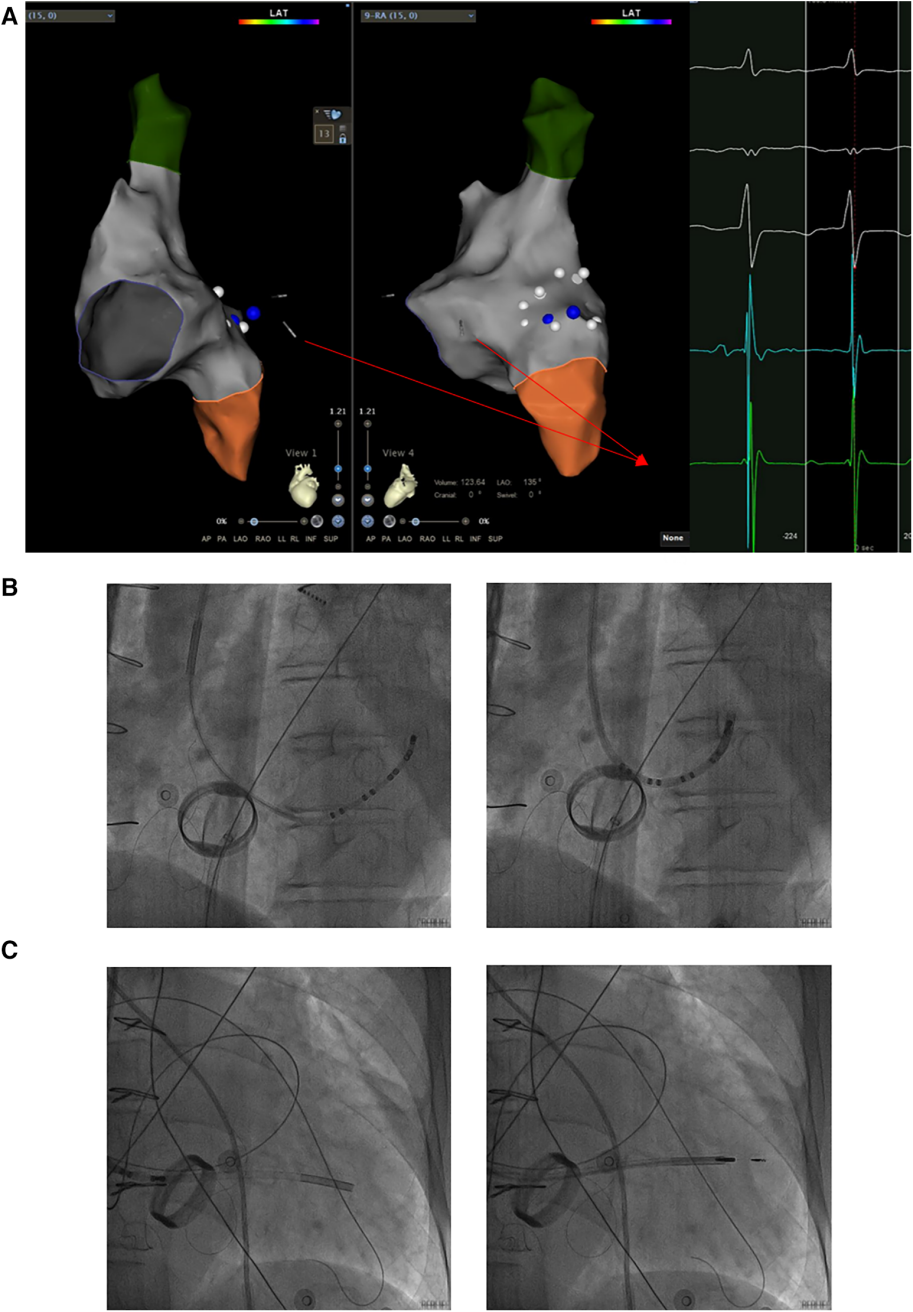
The LV lead was implanted into the LVFW along a sureValve 6250VIS under the guidance of CARTO 3 and x-ray imaging. (A) The CS lead was maneuvered into the LVFW through the mitral valve under the guidance of CARTO 3 and the potential of the CS lead. The potential of the CS lead pointing toward the LVFW is shown in the red box. (B) The CS lead which inserted into the Attain Command + SureValve 6250VIS passed through the centre of FO via the axillary vein followed by under 3D mapping and x-ray system. (C) Along the CS lead, SureValve 6250VIS was placed toward the LVFW. The LV lead was placed along the SureValve 6250VIS and rotated into LVFW. The red arrow shows the potential of the CS lead; the yellow box shows the potential at the center of the FO; the blue box shows the potential at the edge of the FO; LV: left ventricular; LVFW: left ventricular free wall; CS: coronary sinus; FO: fossa ovalis.
